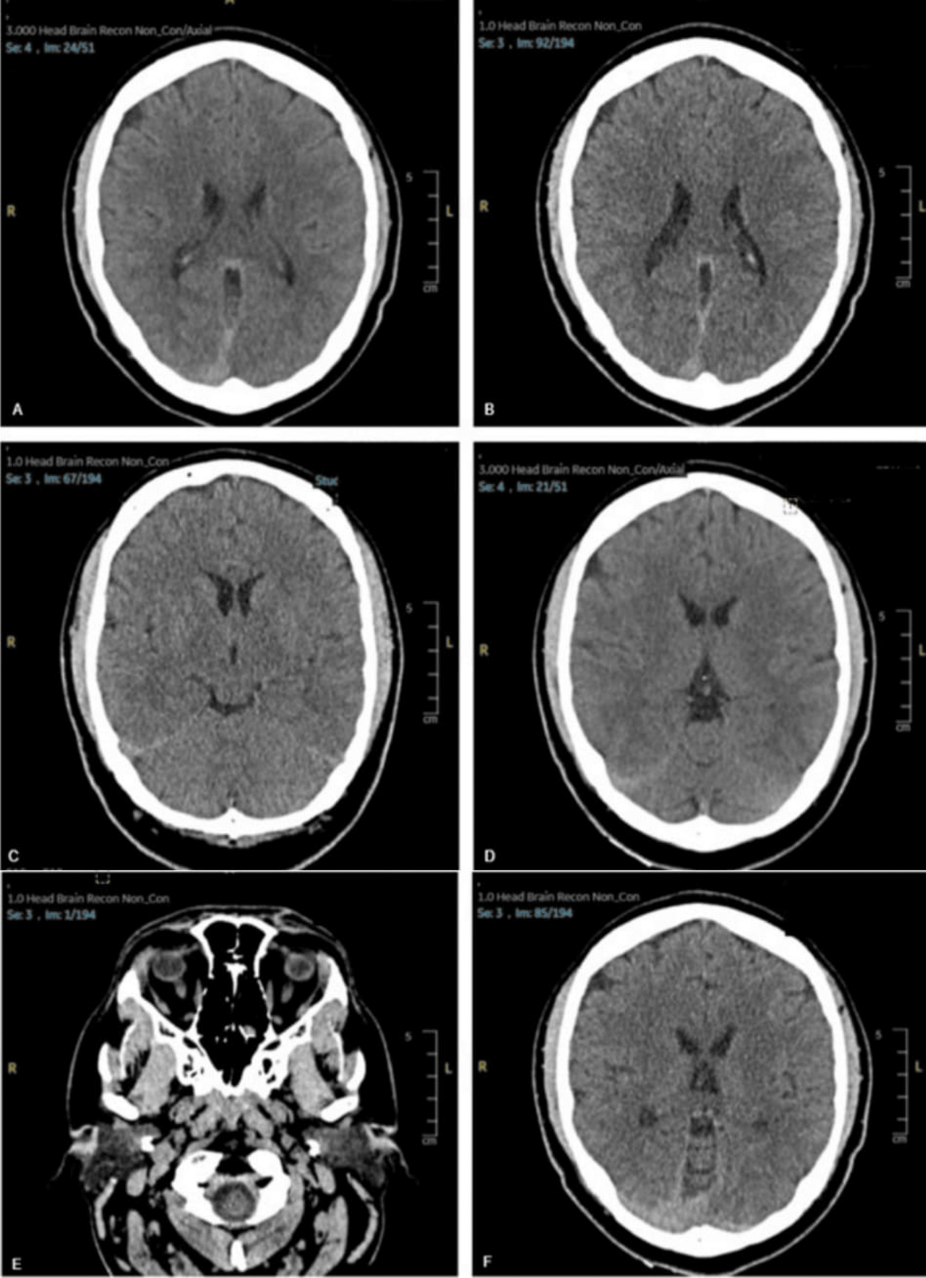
CT Imaging A-F: Images showing various sections of the patient's CT brain.
